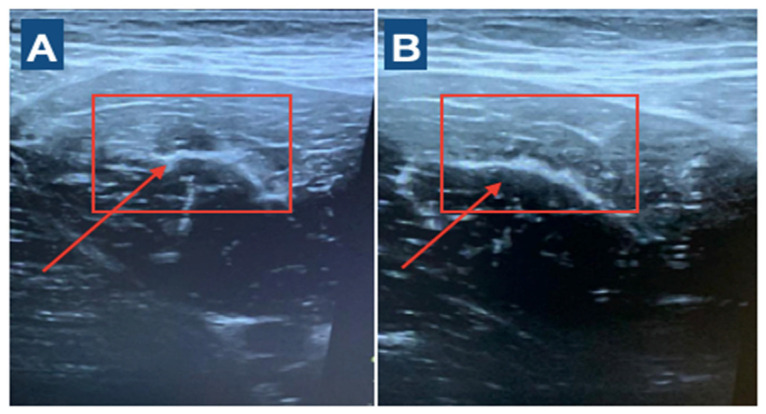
(A). Initial 48 h post-injury. Ultrasound imaging shows muscle fiber discontinuity at the level of tear. (B) Ultrasound imaging at 9 days post-PNE.
